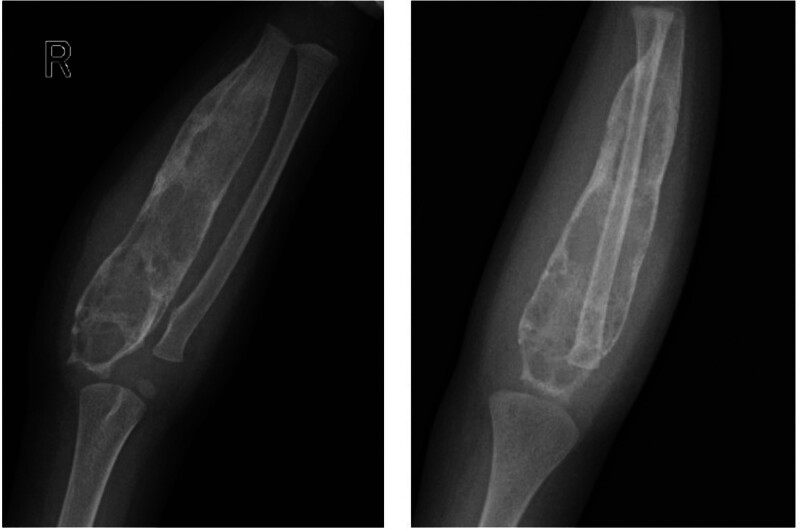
Anteroposterior and lateral X-ray of the ulna depicted the progression of bone expansion in the right ulna, including lateral cortical thinning and disorganized trabecular bone structure, accompanied by high-density shadows indicative of cord-like, patchy, and ground-glass appearances.
